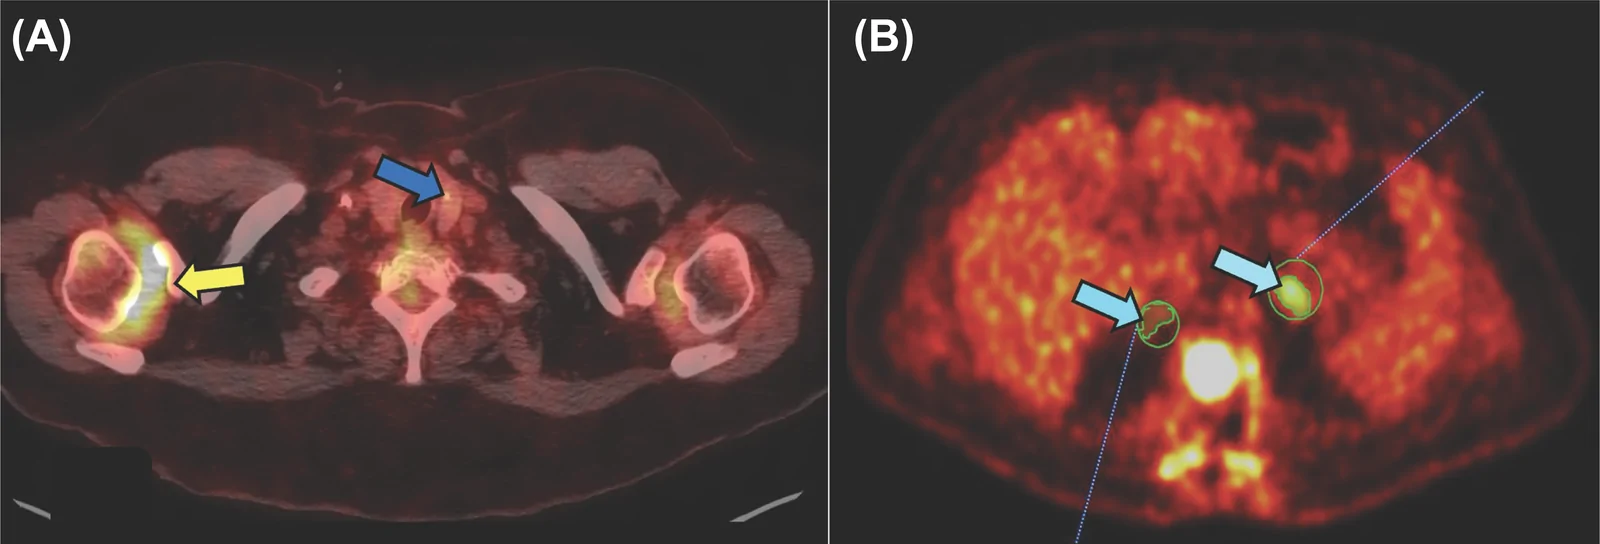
18F-FDG PET/CT. (A) Right shoulder with synovial thickening/effusion and diffuse uptake (SUVmax 7.6), suggestive of synovitis (yellow arrow). Left thyroid nodule with increased uptake (dark-blue arrow). (B) Bilateral adrenal thickening with nodular morphology and right-sided calcification; intense uptake (SUVmax 5.9) (light-blue arrows). Abbreviations: FDG, fluorodeoxyglucose; PET/CT, positron emission tomography/computed tomography; SUVmax, maximum standardized uptake value.
